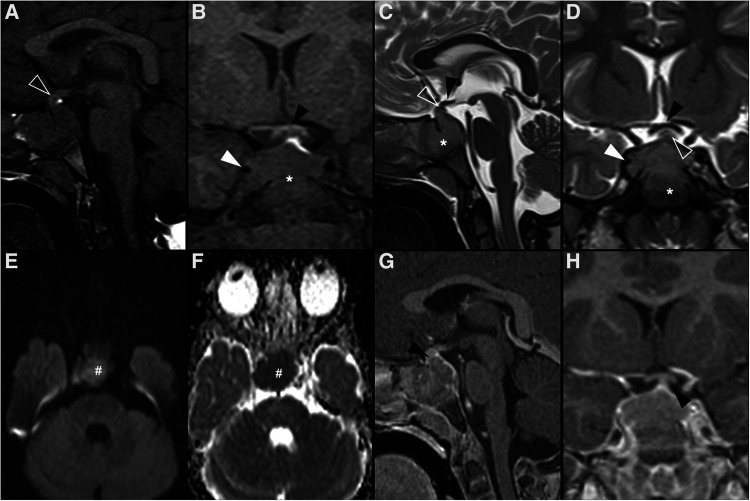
Langerhans cell histiocytosis in a 2-year-old girl presenting with right palpebral ptosis, headache and progressive diminished appetite and activity. Brain MR imaging reveals an expansile mass centered in the sphenoid body and sella turcica, homogeneously isointense to grey matter on coronal (A) and sagittal (B) T1WI, as well as and hyperintense on sagittal (C) and coronal (D) T2WI, mimicking a macroadenoma. The lesion presents suprasellar extension with infundibular thickening (empty white arrowheads), upward bowing of the central optic chiasm (white black arrowheads), as well as signs of clear invasion of the right cavernous sinus with carotid artery stenosis (black white arrowheads). One can clearly depict the preserved T1 hyperintensity of the compressed neurohypophysis in a left suprasellar position (white arrows). There is also destruction of the sellar floor invasion of the sphenoid bone and sinuses (asterisks). Note superior projection of the adenohypophysis and neurohypophysis (empty white arrowhead). Axial DWI (E) and ADC map (F) depict mild restricted diffusion suggestive of hypercellularity (hashtag). Contrast-enhanced sagittal (G) and coronal (H) T1WI reveal mild solid homogeneous enhancement of the lesion. Normal enhancement of the superiorly displaced adenohypophysis is present (black arrowhead). No other parenchymal, leptomeningeal or calvarial lesions were observed.
